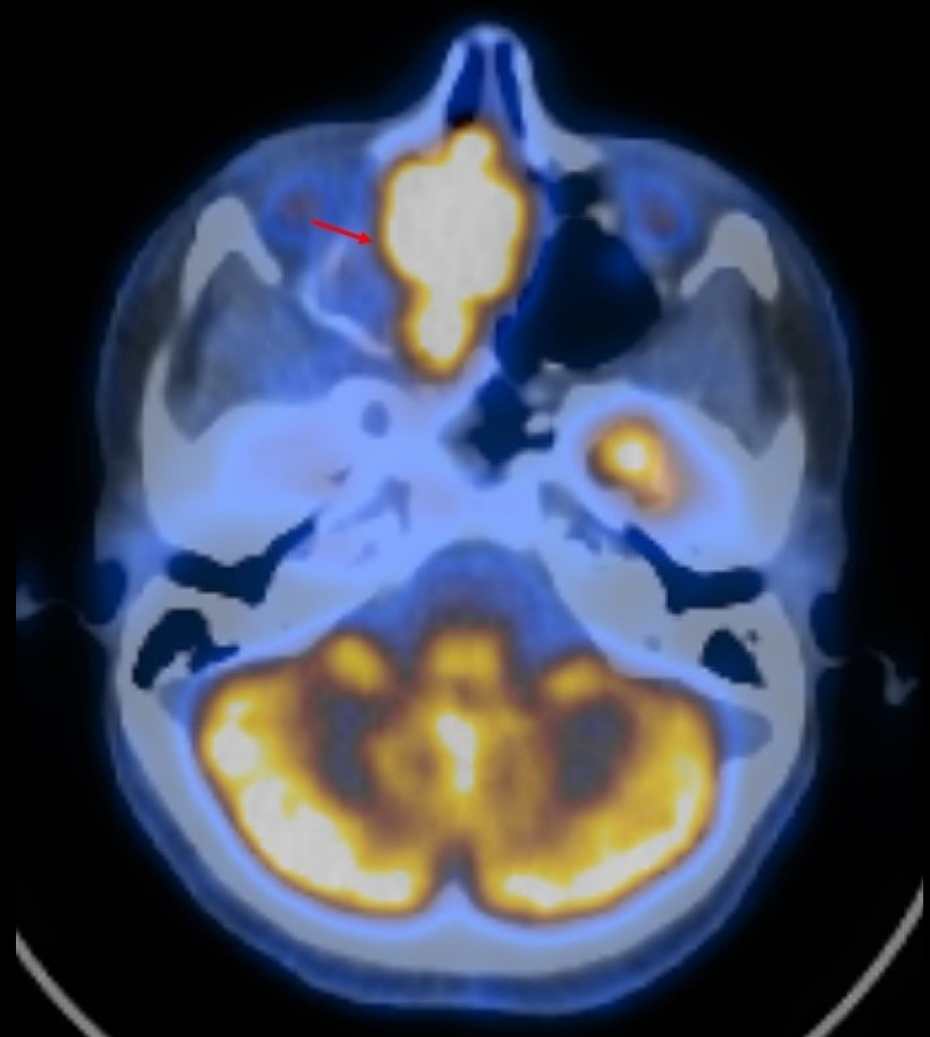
Positron emission tomography/computed tomography (PET/CT) demonstrates high fluorodeoxyglucose (FDG) uptake by the primary neoplasm
